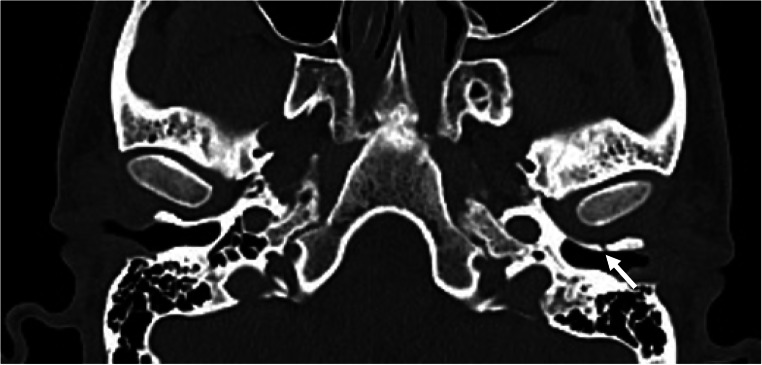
Computed tomography showing isolated left tympanal bone fracture (arrow). The victim received multiple kicks in the head during an assault
